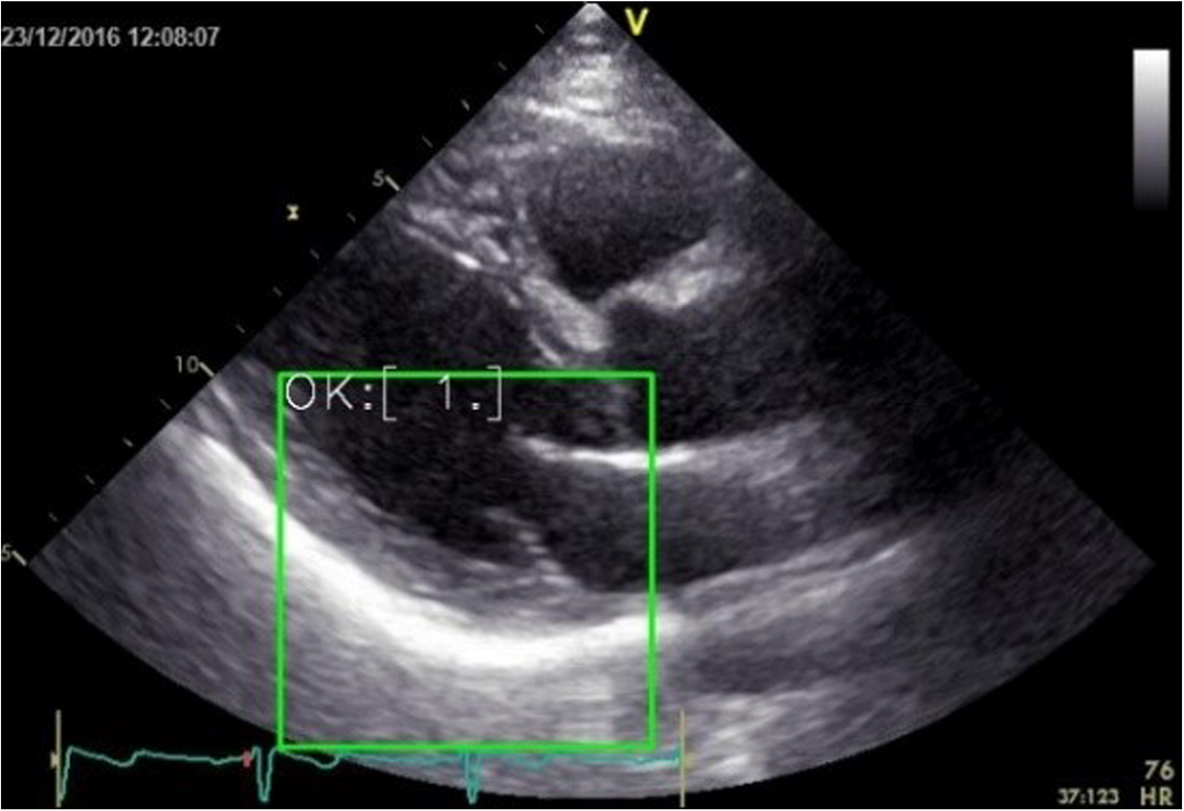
An echocardiogram based on CNN positioning results
